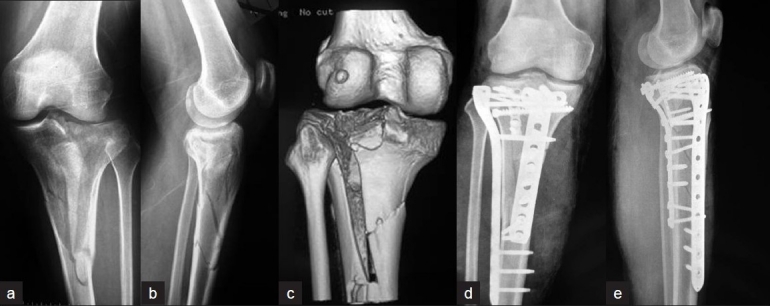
Anteroposterior (a), lateral (b) radiographs, and three-dimensional CT scan (c) of Patient 4 shows the complex fractures of the tibial plateau and metaphysis. Postoperative anteroposterior (d) and lateral (e) X-ray films show the fractures were well reduced and fixed via combined PM and AL approaches. PM= Posteromedial, PL= Posterolateral
